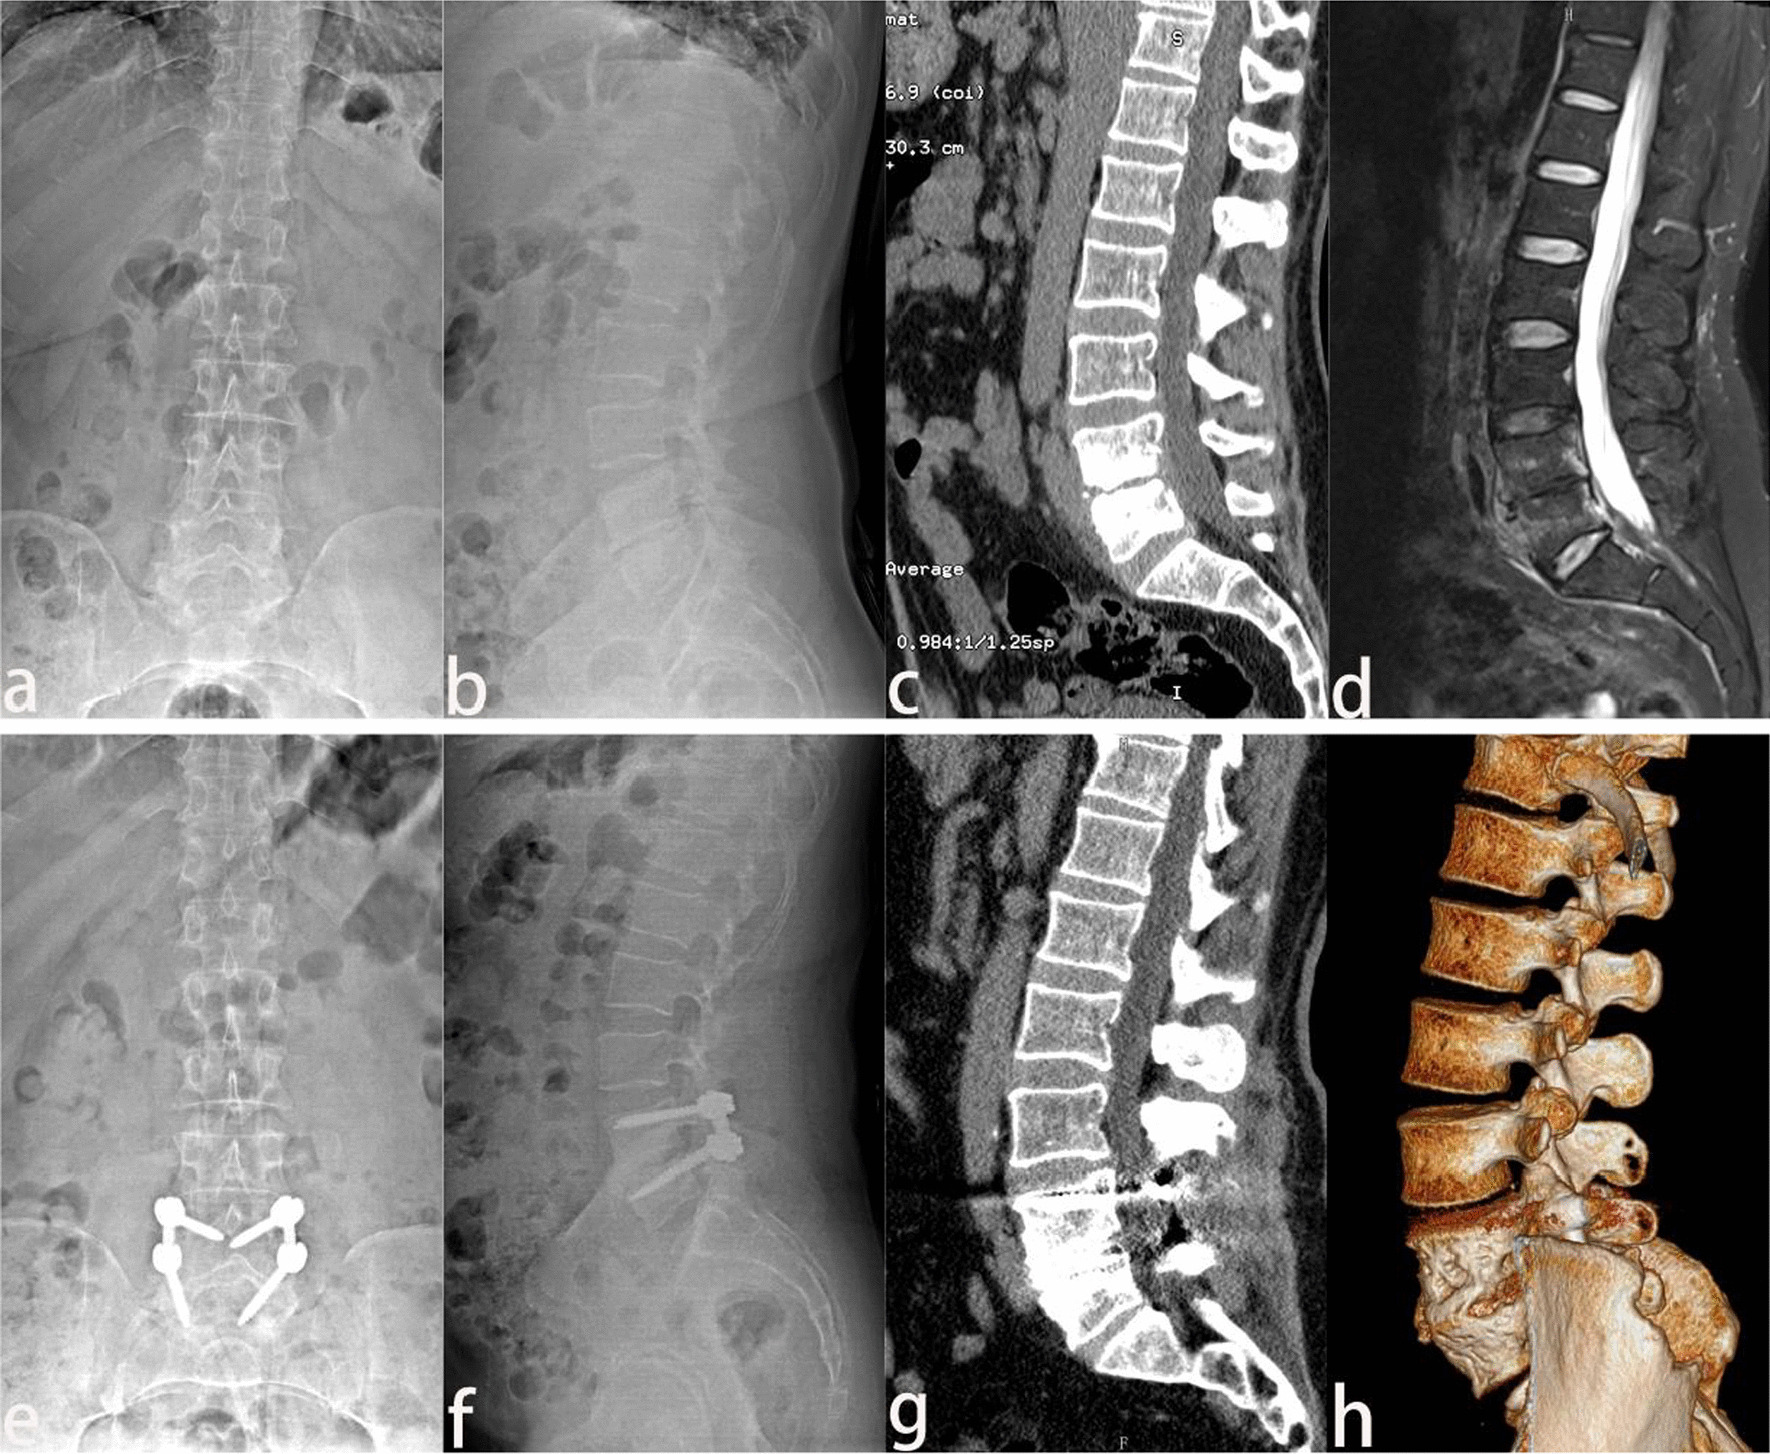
A 57-year-old female with lumbosacral Brucella spondylitis. a–d L5, S1 vertebral body destruction, and intervertebral space narrowing caused by infection were indicated by the anteroposterior and lateral X-ray, CT sagittal reconstruction, and MRI. e, f The vertebral body was fixed firmly by the screw at 3 postoperative months, which was presented by X-ray. g, h CT sagittal and three-dimensional reconstruction demonstrated that the lesion was removed completely, and internal fixation was in a satisfactory position without recurrence of infection at the 6 postoperative months
